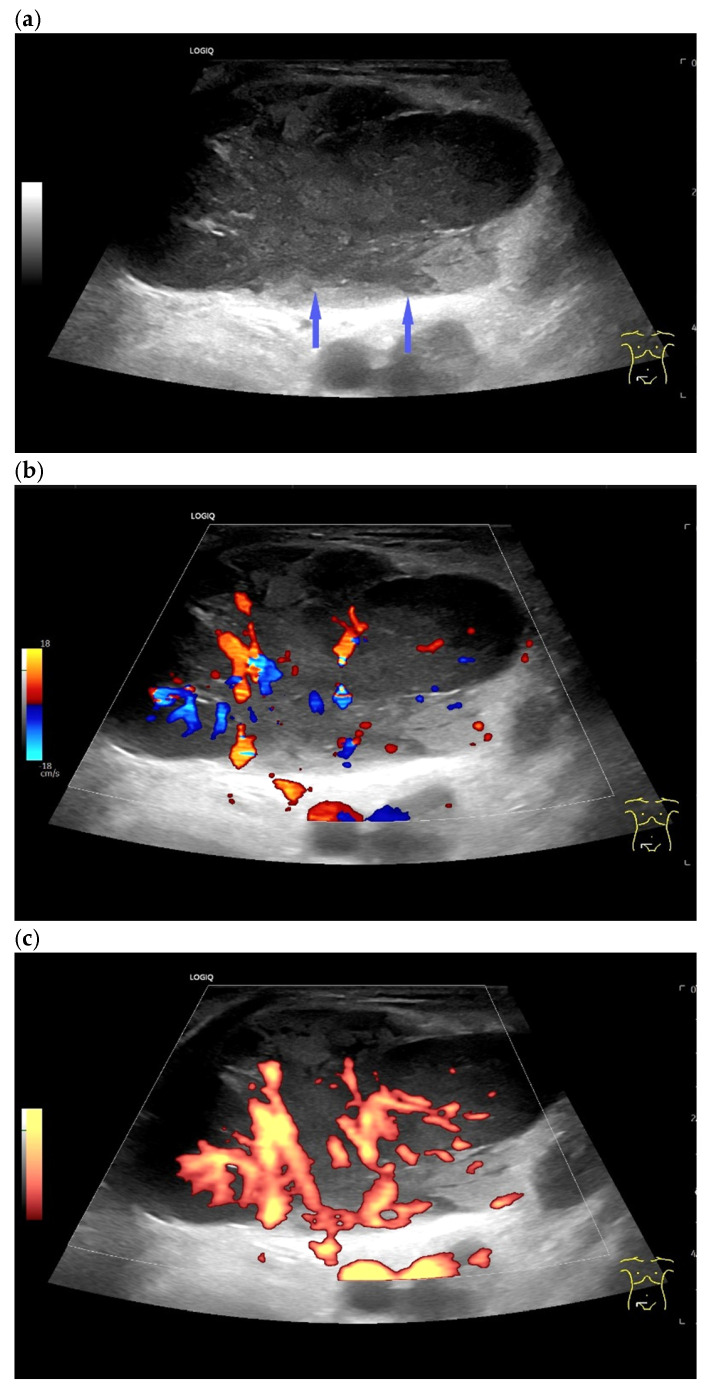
Diffuse large B-cell lymphoma. LN measuring 55 × 35 mm, rounded, very hypoechoic, inhomogeneous. Blurred border (arrows) (a). No central hilum can be identified in color Doppler imaging (b). Power Doppler shows two feeding vessels on the longitudinal side, which branch irregularly (c).
